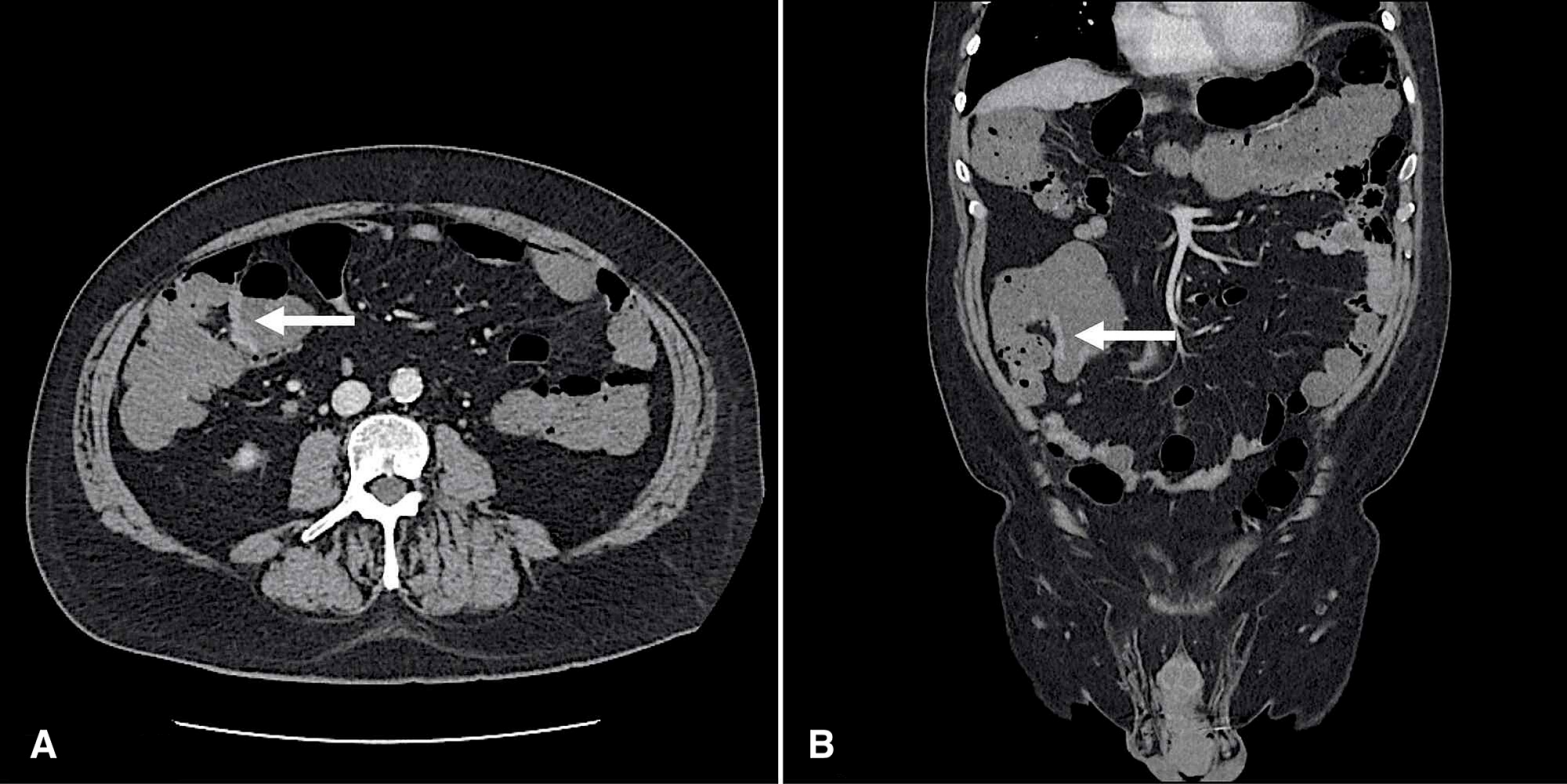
Computed tomography of the abdomen and pelvis with contrast Axial cross-section (A) and coronal cross-section (B) demonstrating focal asymmetric wall thickening and enhancement (white arrow) along the right lateral margin of the cecum measuring 5 mm in thickness concerning for potential colon cancer. The remainder of the gastrointestinal tract with normal wall thickness without any other surrounding inflammatory changes or visible paracolic adenopathy.
